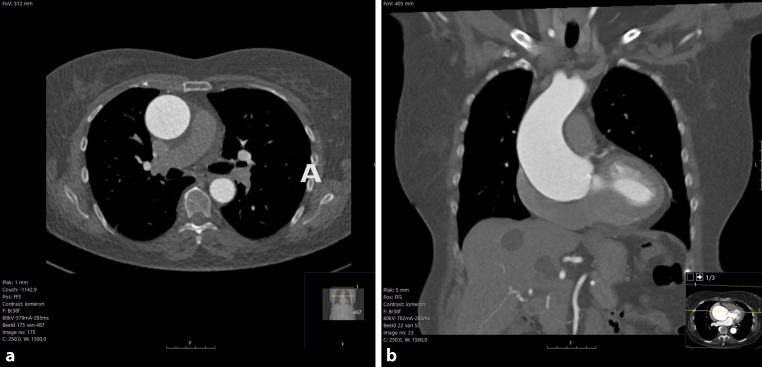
a, b CT-scan of the aorta prior to symptom onset with dilated aortic root and ascending aorta
